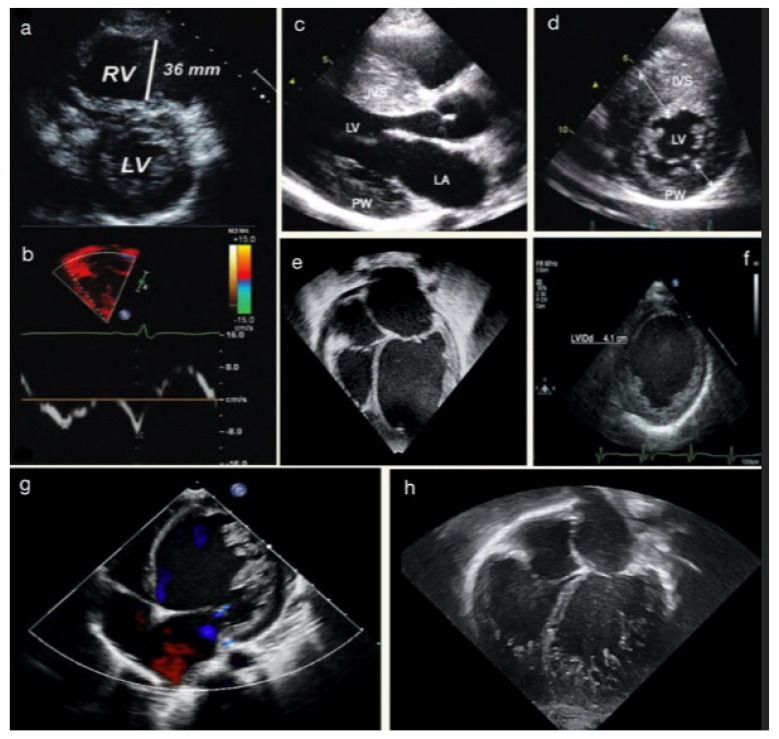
(a) Parasternal short-axis view in a 14-year-old female with ventricular tachycardia during exercise, demonstrating right ventricular (RV) enlargement with suspected ACM. (b) TDI of the RV free-wall showing diminished tricuspid annular TD velocities of the same patient. (c) Parasternal long-axis view in a 13-year-old male with SIV hypertrophy in HCM. (d) Parasternal short-axis view showing hypertrophy of mid-ventricular IVS. (e) Apical-4-chamber view showing dilated LV and LA in suspected DCM. (f) Parasternal short-axis view demonstrates LVEDD at the papillary muscles level. The images (g,h) show two different cases of LVNC.
